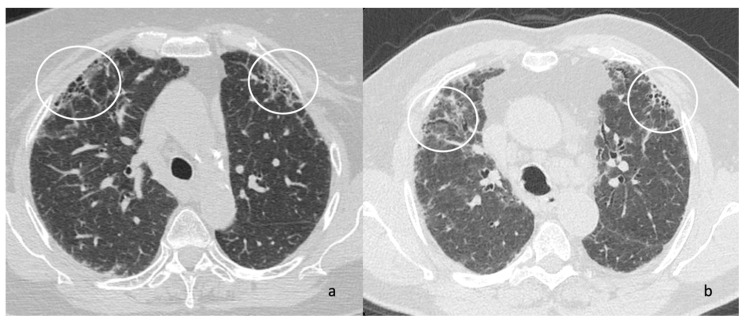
High-resolution computed tomography (HRCT), axial images (a,b). Predominantly distribution in the ventral-anterior regions of both upper lobes—as clearly demonstrated on Figure 3a,b (white circles).
